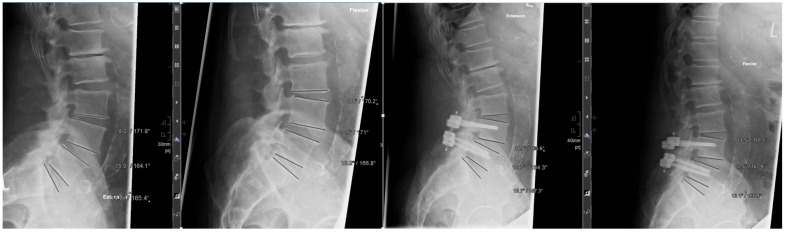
Extension/flexion X-rays with measurements of the segmental angle.
